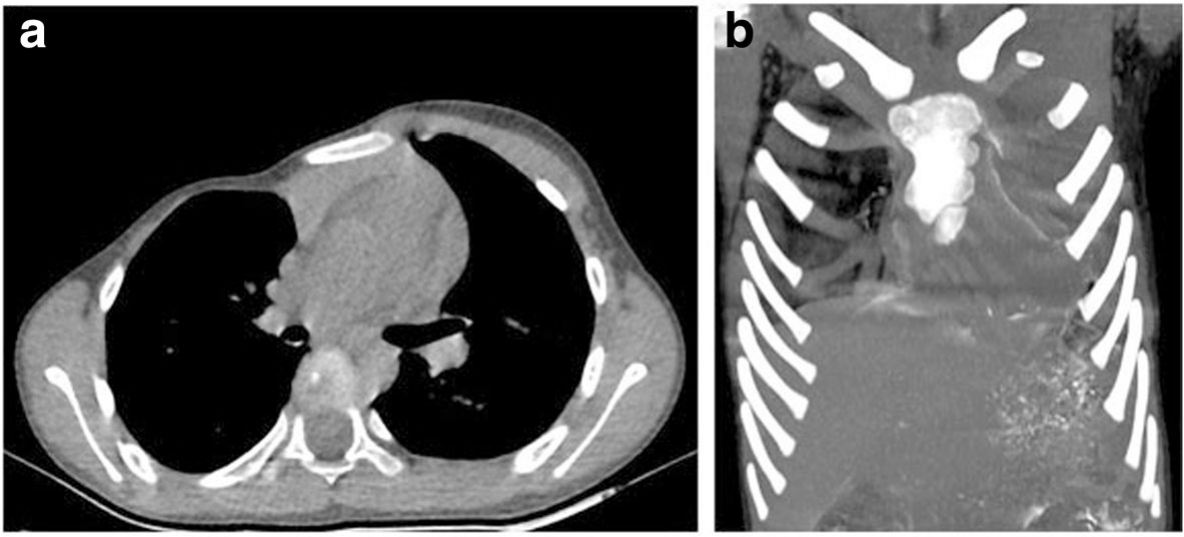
High resolution computed tomography of three-dimensional reconstruction of chest. a Depression of the sternum which was rotated to the right and absence of the right chest wall soft tissue. b Multiple ribs abnormally extended on right side, particularly from the right 3rd to 7th rib, parasternal absence of the right partial 3rd rib cartilage(arrowheads)
